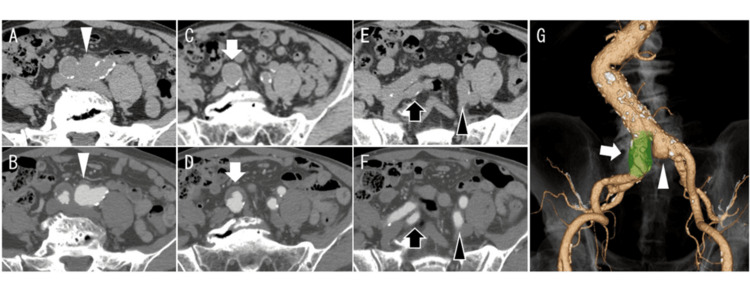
Preoperative CT Panels A, C, and E show unenhanced (plain) CT images, while panels B, D, and F show contrast-enhanced CT images. (A and B) Saccular left common iliac artery aneurysm with a maximum diameter of 28 mm (white arrowhead). (C and D) Right common iliac artery aneurysm with a maximum diameter of 24 mm (white arrow). (E and F) No aneurysmal enlargement is observed in the bilateral internal iliac arteries (black arrow: right side; black arrowhead: left side). (G) Three-dimensional reconstruction image showing the left saccular and right fusiform common iliac artery aneurysms (white arrowhead and white arrow, respectively). CT: computed tomography
